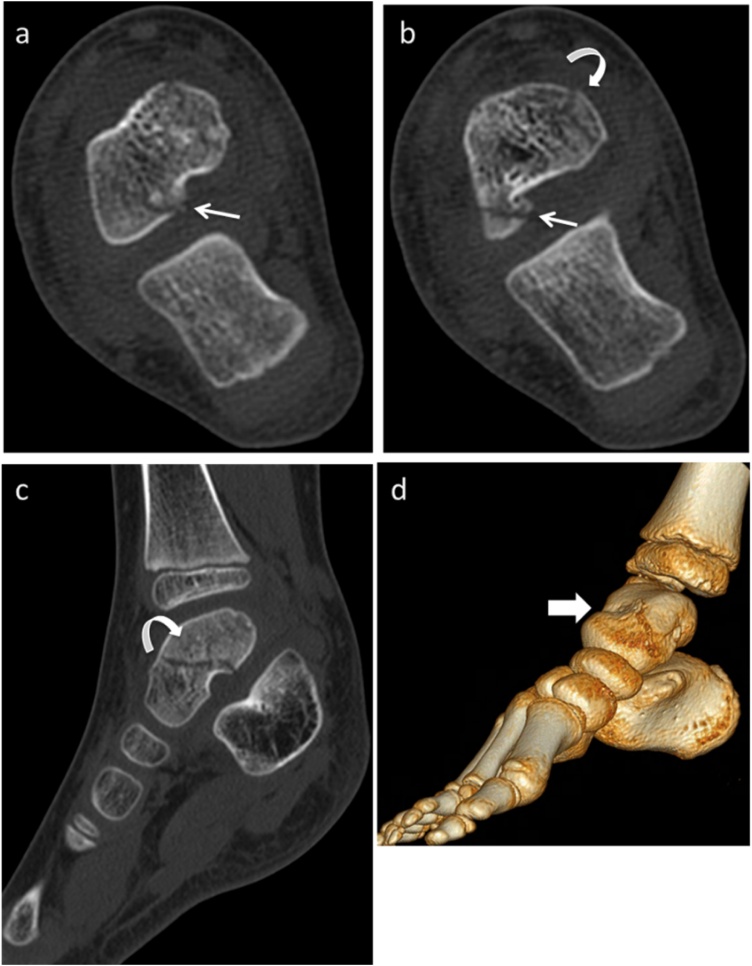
a and b. Axial CT scan shows multifragmentary fracture of the body of the astragalus (curved arrow) with intraarticular component (thin arrow). c. Sagittal reconstruction with hypodense traces of the talus body (curved arrow). d. Volumetric reconstruction shows the fracture of the talus body (arrow).
